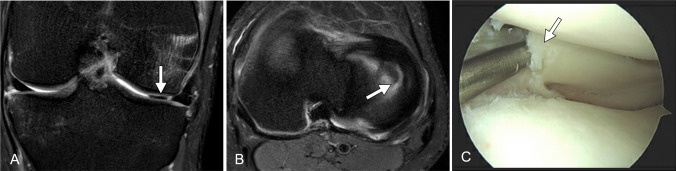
Vertical flap tear (historically termed a “parrot-beak” tear at arthroscopy) in a 25-year-old man with ACL tear. Coronal proton-density-weighted fat-suppressed MRI (A), axial proton-density-weighted fat-suppressed MRI (B), and arthroscopic view (C) depict a radial oblique configuration of tear involving the body and anterior horn of the lateral meniscus with a displaced meniscal flap (arrows) along the free edge
